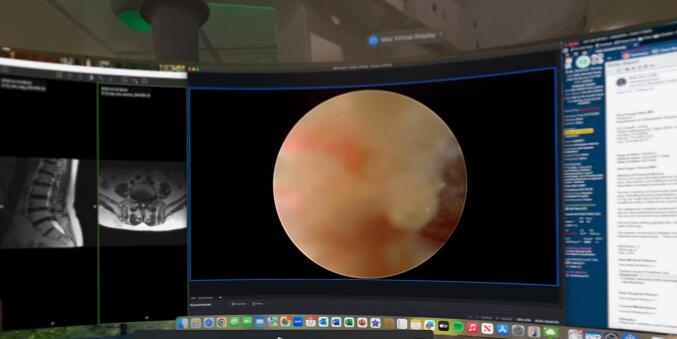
Intraoperative photograph depicting the endoscopic video in the center of the AR view, the preoperative MRI on the left of the AR view, and the clinical information from the EMR on the right of the AR view.
